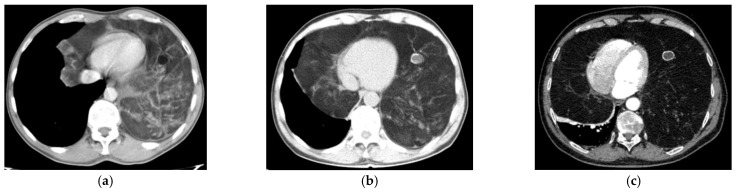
CT scan from the same presentations in 2008 (a), 2018 (b), and 2023 (c), revealing the large mediastinal mass consisting of inhomogeneous tissue with fatty densities, fibrous trabeculae, and intratumorally calcification.
